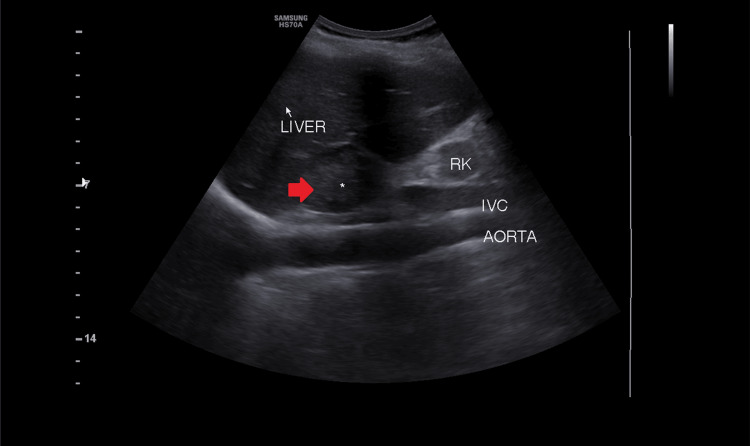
Ultrasonography of the abdomen showing a well-defined heterogeneously hypodense lesion (red arrow) measuring 36 mm x 32 mm in the right suprarenal region indenting right lobe of liver. Red arrow pointing to hypodense mass over the right kidney. RK: right kidney; IVC: inferior vena cava; mm: millimeter
